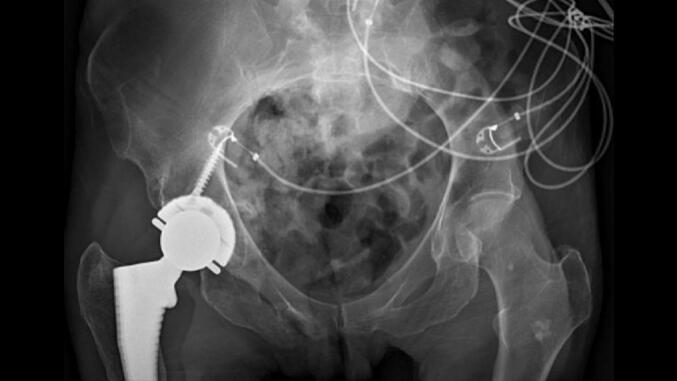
AP radiographic image of pelvis showing right revision total hip arthroplasty with constrained liner.
